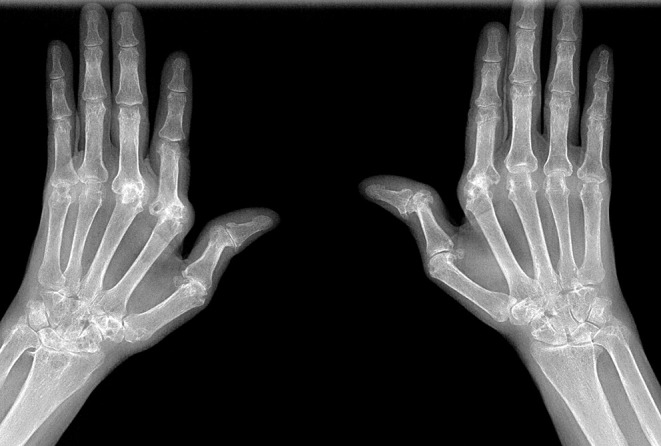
X-ray of the hands showing joint deformities with surrounding osteopenia.
